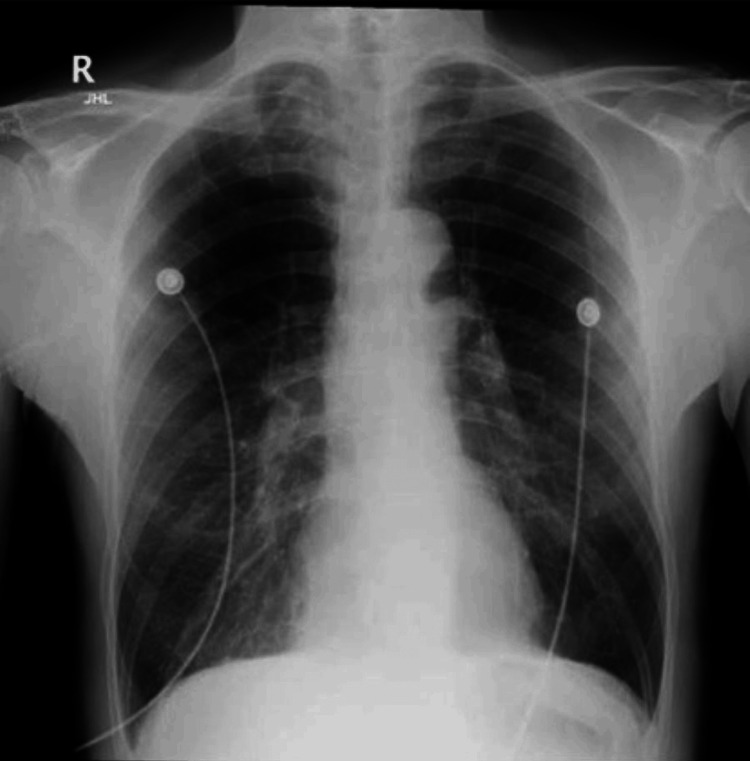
Chest radiograph in Case 1 demonstrating hyperinflation and diaphragmatic flattening An anteroposterior chest radiograph demonstrates hyperinflated lung fields with diaphragmatic flattening, supporting obstructive lung physiology in a patient with childhood-onset asthma, prior tobacco exposure, and emphysematous disease.
